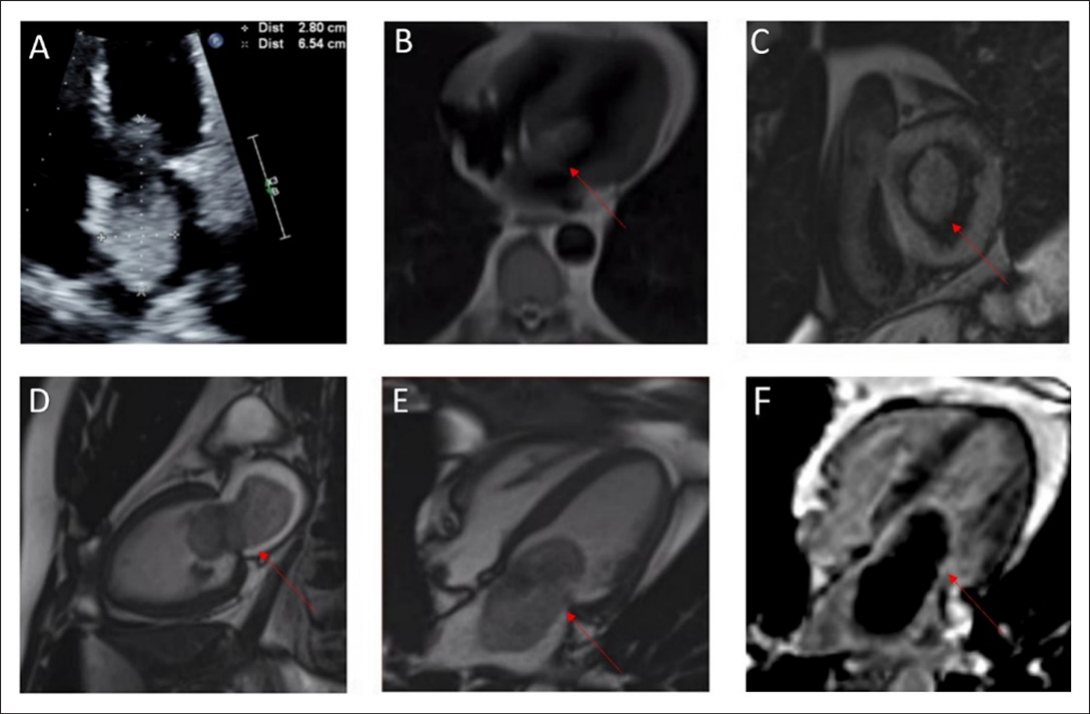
(A) Transthoracic echocardiogram of normal LV systolic function with an ejection fraction of 55% to 60% and large mobile echo density in the left atrium attached to the atrial septum; (B-E) cardiac magnetic resonance (CMR) imaging obtained for further characterization of the cardiac mass confirmed the presence of a large atrial mass attached to the fossa ovalis; (F) delayed gadolinium enhancement CMR with long inversion time suggested a thrombus.
